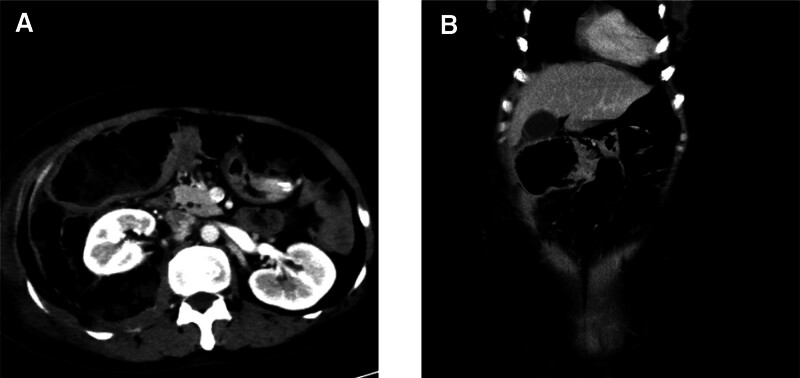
Abdominal computed tomography. (A) Transverse plane of colon mass and (B) coronal plane of colon mass.
